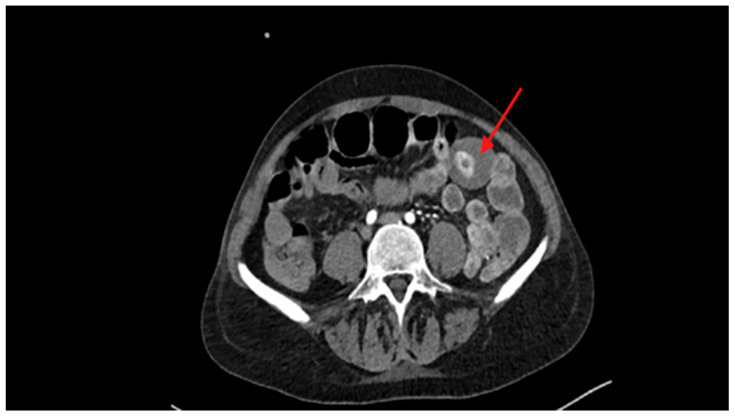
CT scan identified an intensely hyperdense endoluminal spot in the small intestine (red arrow).
